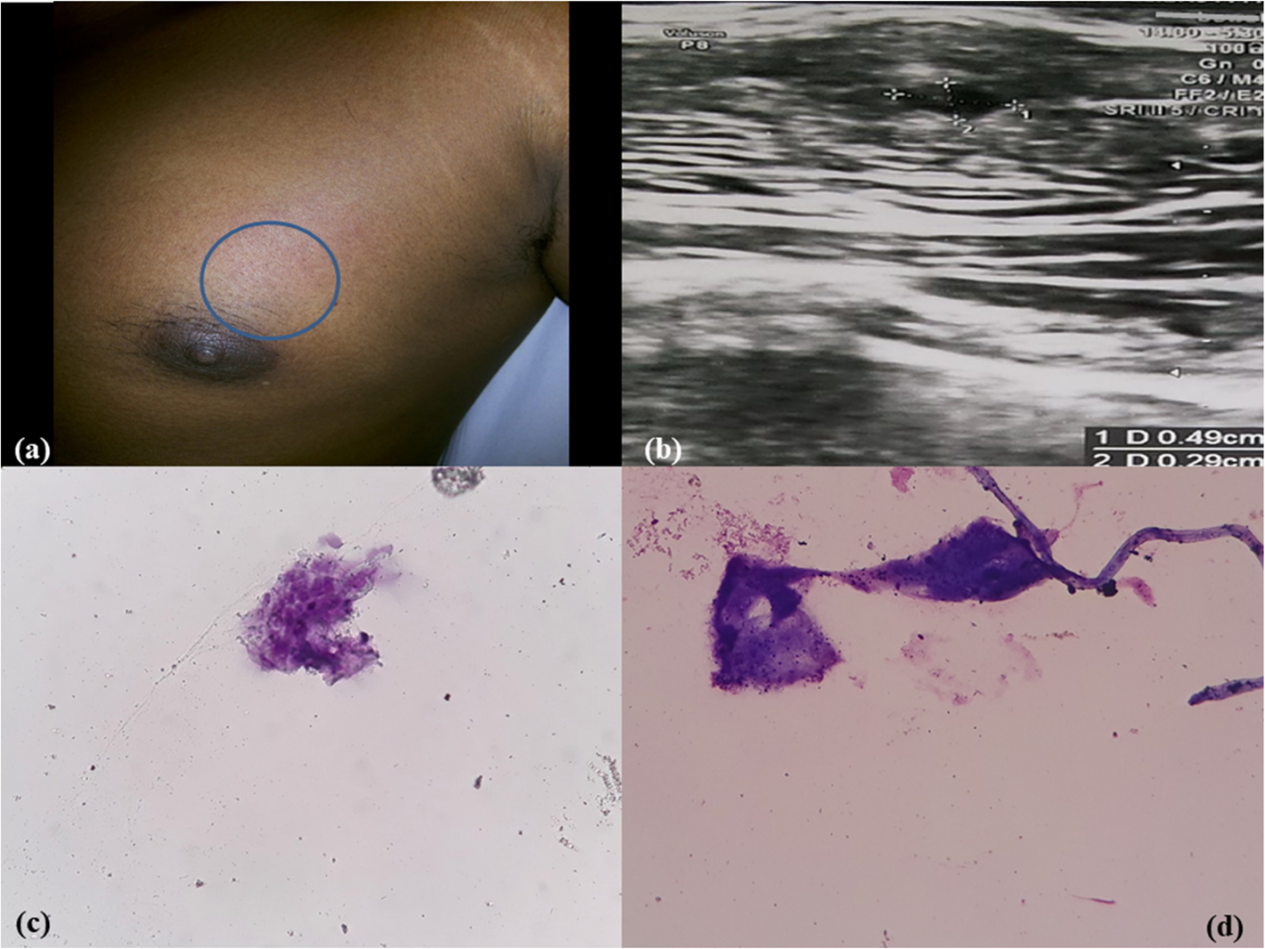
Case 1. a, b Swelling in left chest wall. Ultrasonography showed a small cystic lesion of 0.5 × 05 mm in left chest wall. c, d Cytology revealed a fragment of parasite intestine and degenerated remnants of parasite with inflammatory background. (Toluidine blue, × 10)
